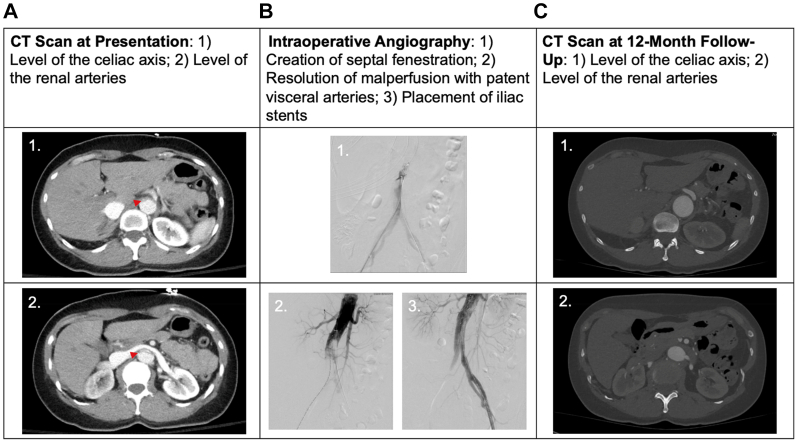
(A-C) Case 1. A 44-year-old woman with Marfan syndrome and Society for Vascular Surgery/Society of Thoracic Surgeons (SVS/STS) type A_10B_. *Red arrow*, true lumen (TL). *CT*, Computed tomography.
